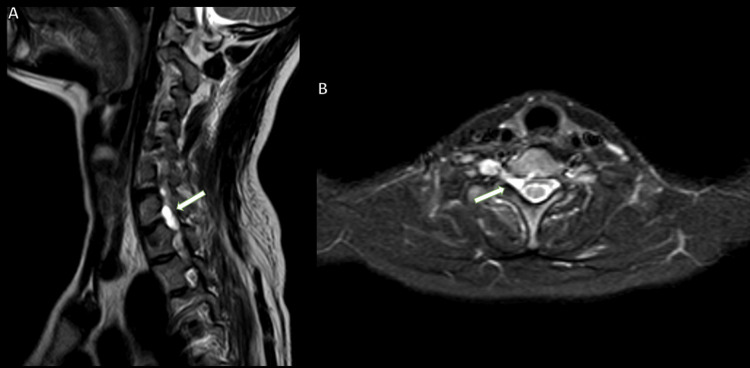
MRI-brachial plexus (A) T2 sagittal section, (B) STIR axial section of case 3 MRI: Magnetic resonance imaging; STIR: short tau inversion recovery; CSF: cerebrospinal fluid MRI-brachial plexus (A) T2 sagittal section shows the presence of extradural, multifocal collections that closely resemble the CSF within the spinal canal, situated on both the right dorsal and ventral sides. (B) STIR axial section shows these collections extend into the right neural foramen at the levels between the C5-C6 and C7-T1 vertebrae. Notably, these collections lack central neural elements and are characterized by margins that transition from smooth to lobulated. This results in mild compression and a slight leftward displacement of the cervical cord segments (large thick white arrow).
